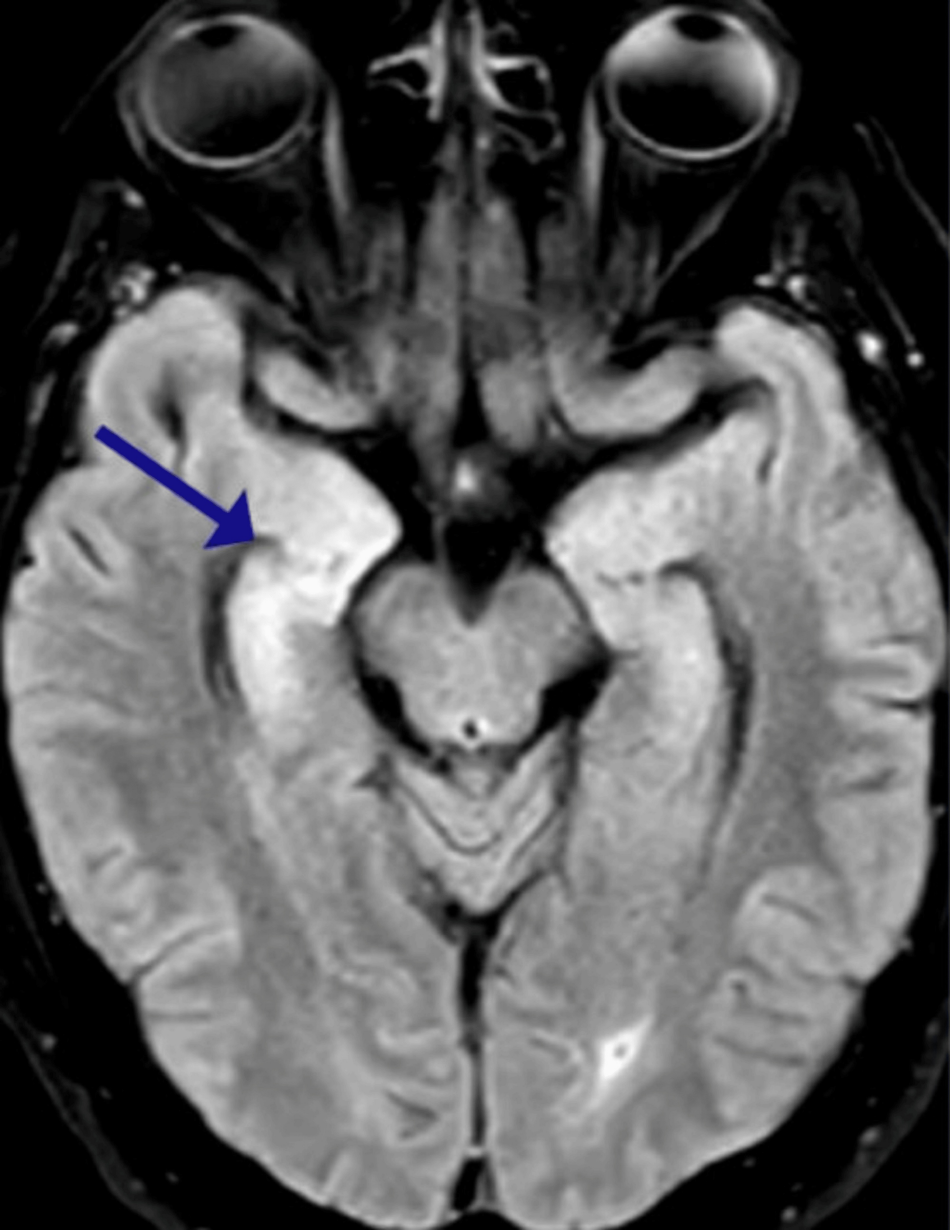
Brain MRI - axial T2 FLAIR sequence Bilateral, right (blue arrow) greater than left, T2 FLAIR hyperintensity seen on mesial temporal lobe and hippocampus FLAIR, fluid-attenuated inversion recovery; MRI, magnetic resonance imaging
